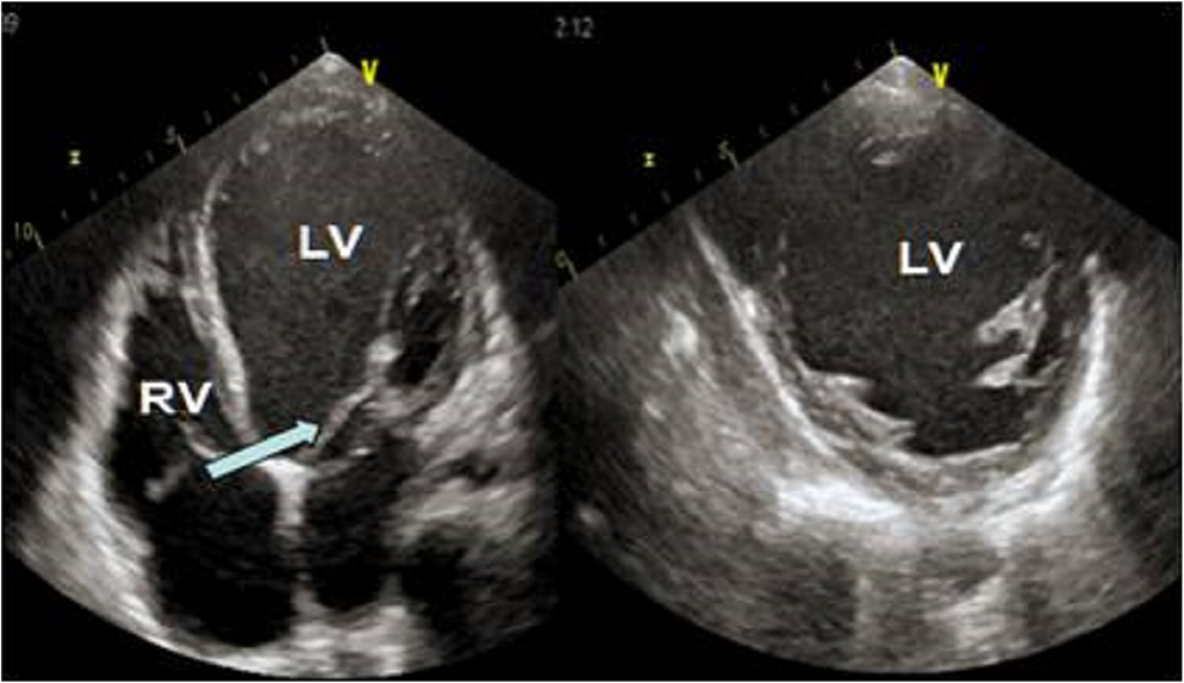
Pre-implantation echocardiographic images. Dilatation of the left ventricle and right ventricle, results of mitral valvuloplasty (*arrow*). *LV* left ventricle, *RV* right ventricle
